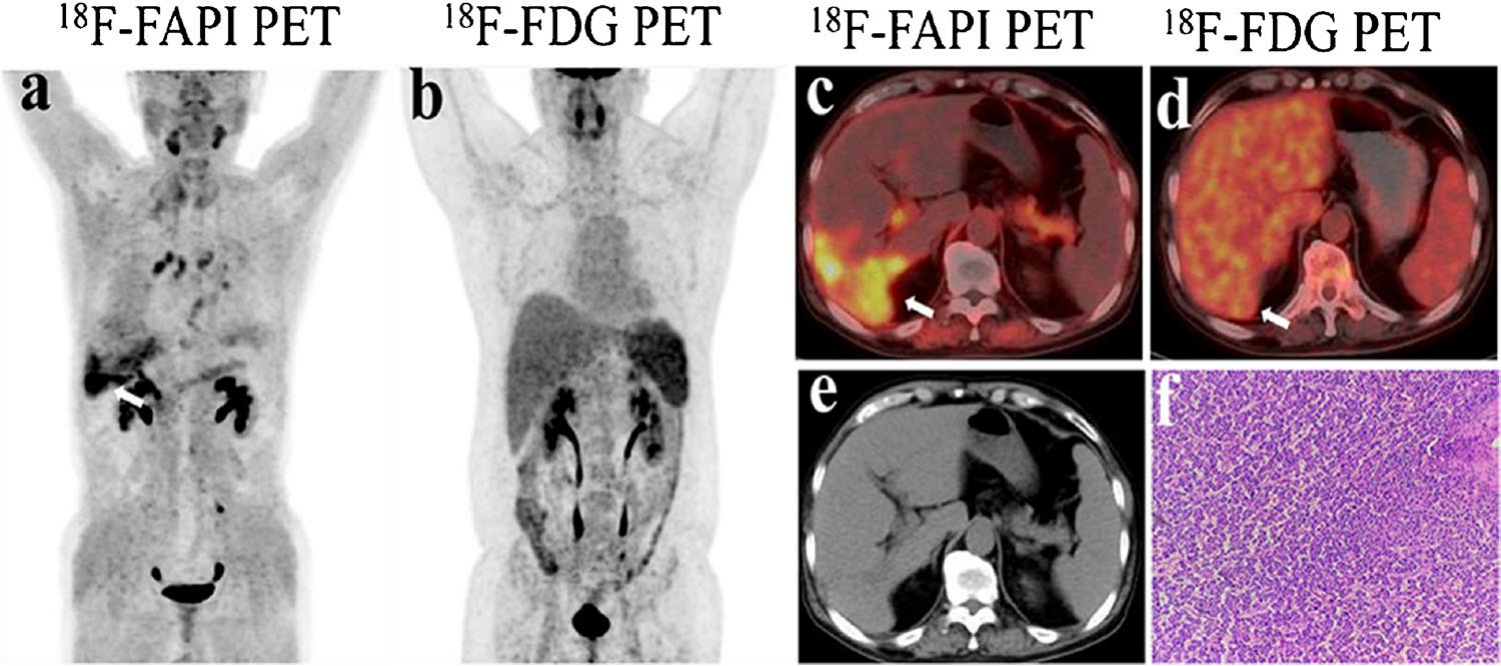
A 52-year-old male with liver discomfort underwent both ^18^F-FAPI-04 PET/CT and ^18^F-FDG PET/CT imaging for initial assessment. a, c
